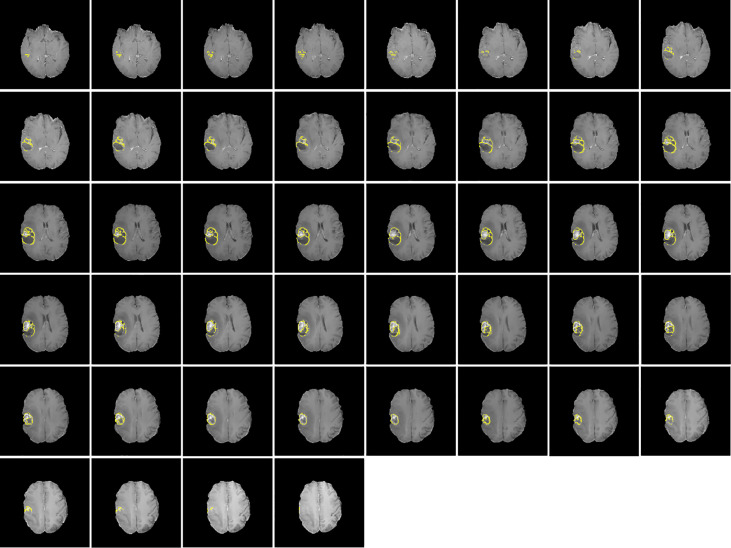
Contoured ROIs (yellow) showing GBM on contrast enhanced T1-weighted MR images. Adapted from CBICA Image Processing Portal; https://ipp.cbica.upenn.edu/. A web accessible platform for imaging analytics; Center for Biomedical Image Computing and Analytics, University of Pennsylvania.
